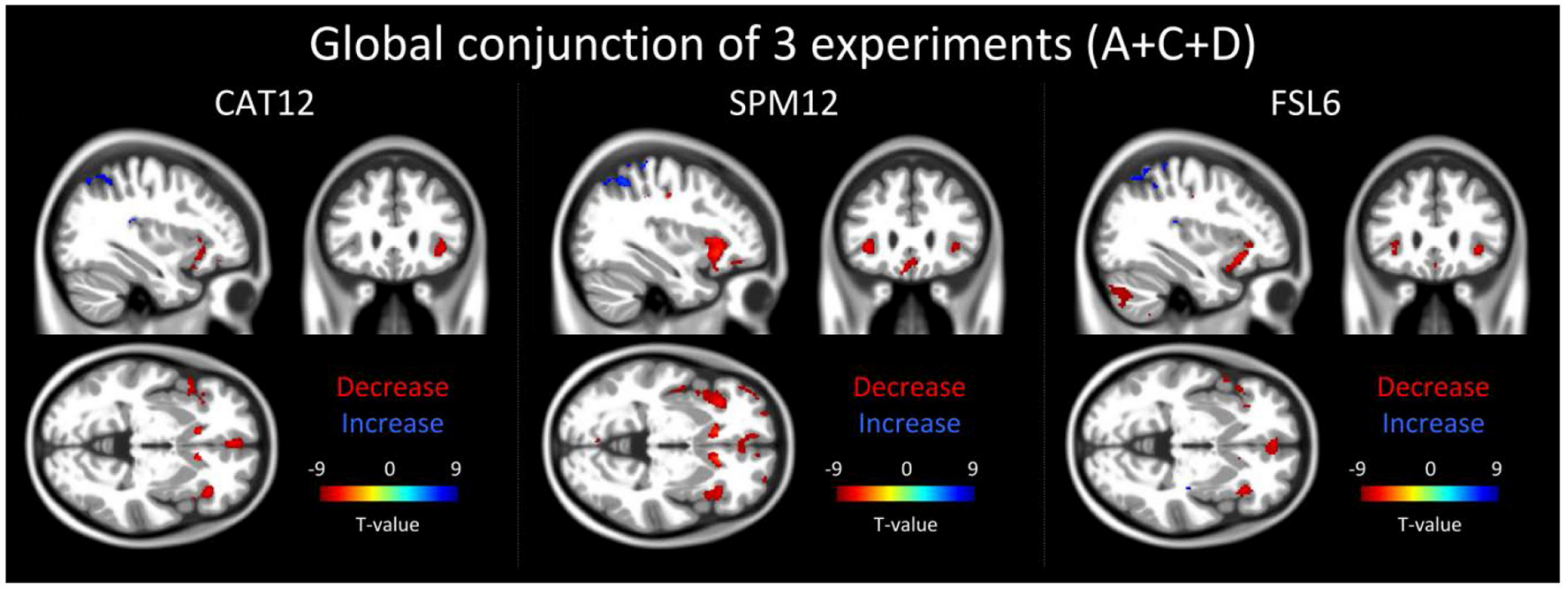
The result of the global conjunction test across all three reported experiments (20 participants for each experiment) is shown in 3 orthogonal slices (xyz−34 27−2 mm) for three different software packages: CAT12, SPM12 and FSL6. Global conjunction tests where the differences between the time points were consistently high and jointly significant across all three experiments (A+C+D) using a minimum statistic. Decreases in gray matter of structural magnetic resonance images are displayed in red colors while increases are indicated by blue colors.
